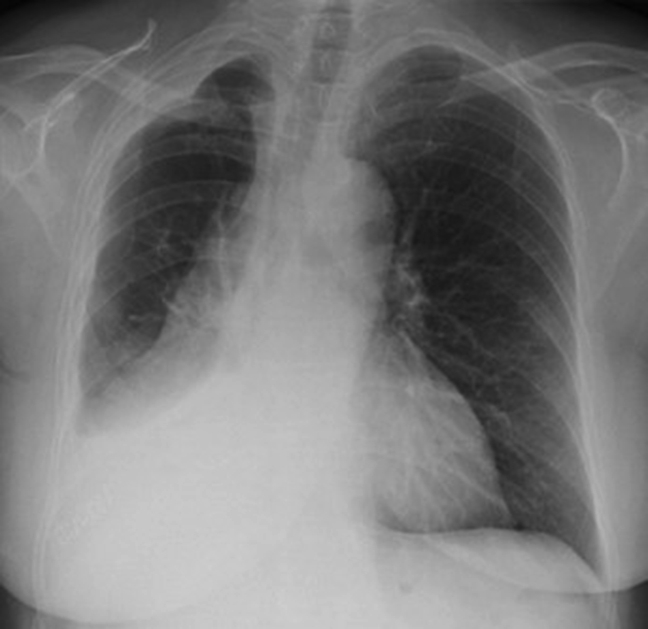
Chest radiographic images after a right re-redo video-assisted thoracoscopic surgery lower lobectomy with middle lobe preservation.
